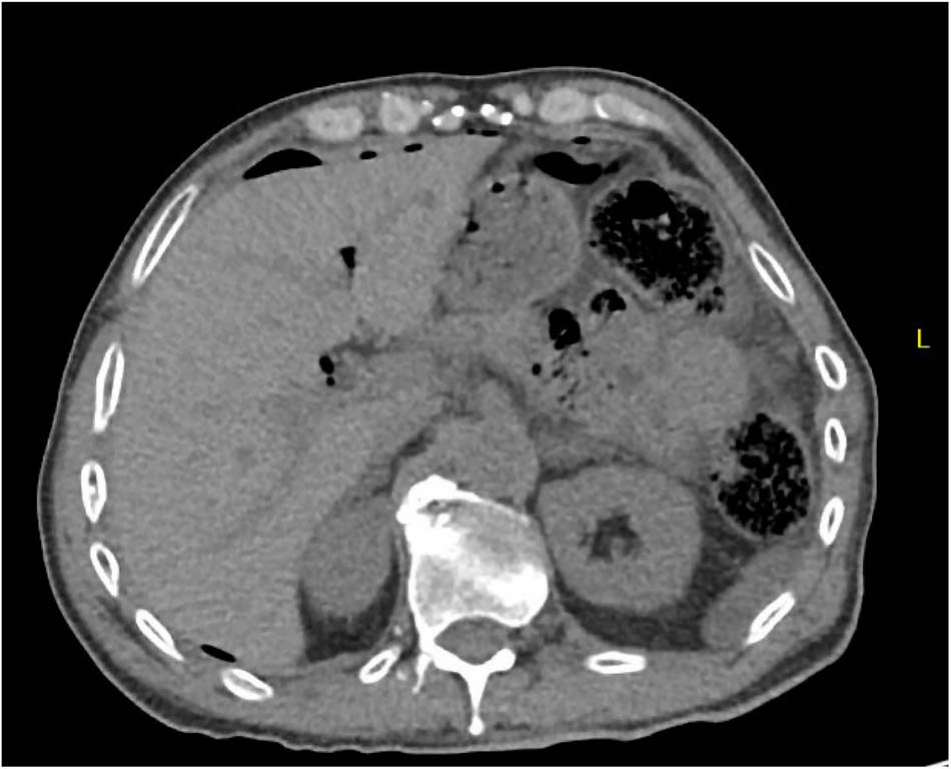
Patient C. Initial CT abdomen and pelvis showing free air concerning for perforated hollow viscus.
